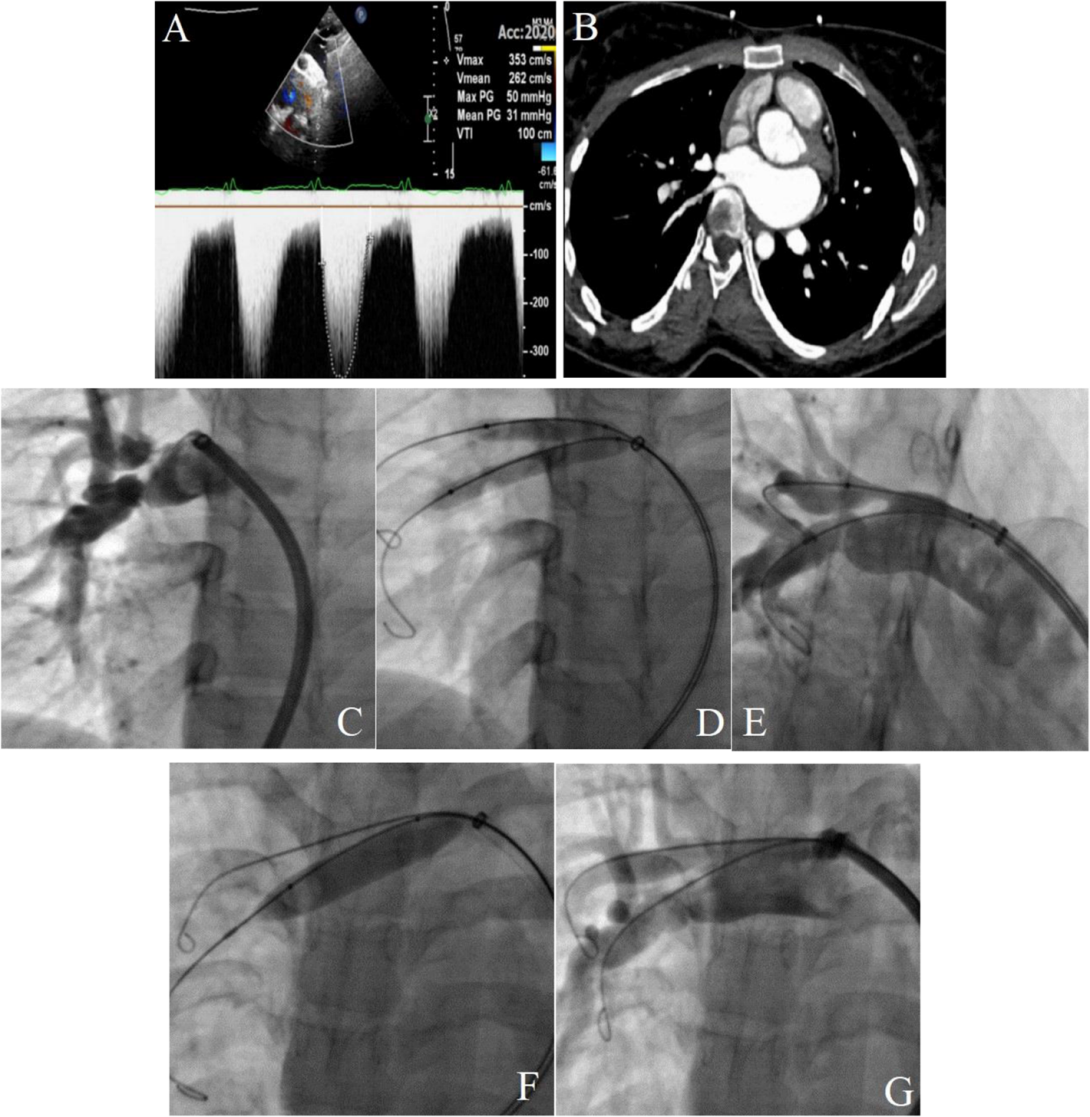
(A and B) Transthoracic echocardiography depicts a significant gradient at the origin of the right pulmonary artery (A). Pulmonary computed tomography angiography reveals a significant stenosis at the origin of the right pulmonary artery (B). (C, D, E, F, and G) Selective right pulmonary artery injection shows significant stenosis in the upper and lower lobar branches (C). Kissing balloon dilation of both branches is done (D). Injection after balloon percutaneous angioplasty shows residual stenosis in both branches (E). Separate and simultaneous balloon percutaneous angioplasty procedures are performed on both branches using larger balloons (F). Final angiography shows mild residual stenosis in the lower branches with acceptable blood flow in the distal branches (G)
